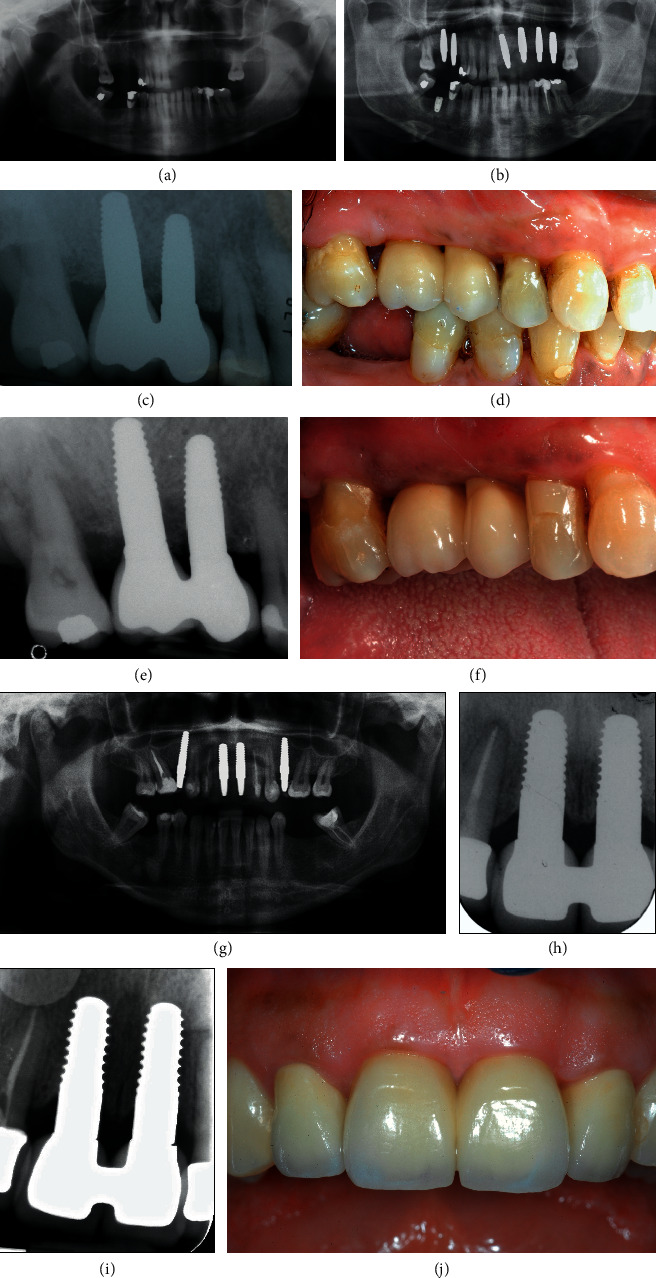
(a) Presurgery OPT. (b) Postsurgery OPT. (c) One-year follow-up RX. (d) Clinical situation at 1-year follow-up. (e) 10-year follow-up RX. (f) Clinical situation at 10-year follow-up. (g) Postsurgery radiographic situation. (h) Radiographic situation at 1 year. (i) Radiographic situation at 10 years. (j) Clinical situation at 10 years.
